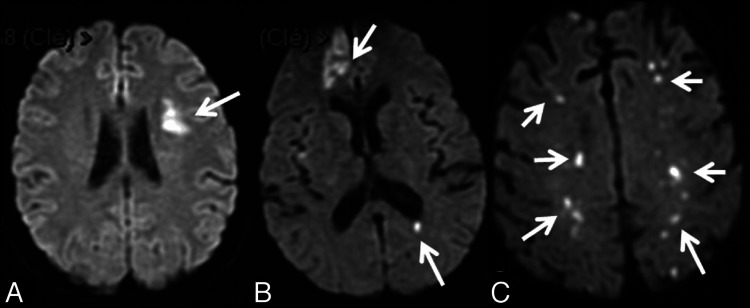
DWI MRI showing acute ischemic lesions in three different patients (A) Single territorial infarct; (B) Territorial plus small cortical/subcortical infarcts; (C) Multiple small watershed ischemic lesions (arrows). Image credit: Hess et al. [14] MRI, magnetic resonance imaging; DWI, diffusion-weighted imaging
